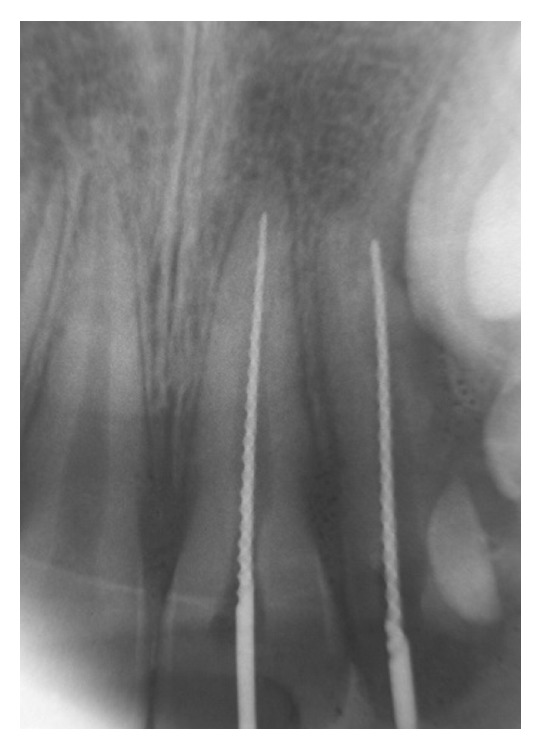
Periapical radiograph of the initial exploration of root canals (first session; March 1998).
